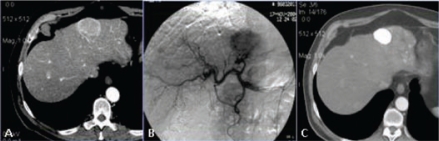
(a) pre-TACE CT shows a mild hypervascularity around the NET liver metastasis to be treated; (b) hepatic artery angiogram confirms the lesion, fed by the left hepatic artery; (c) post-TACE CT shows the good result after treatment, with a homogeneous Lipiodol uptake and an initial shrinkage.
